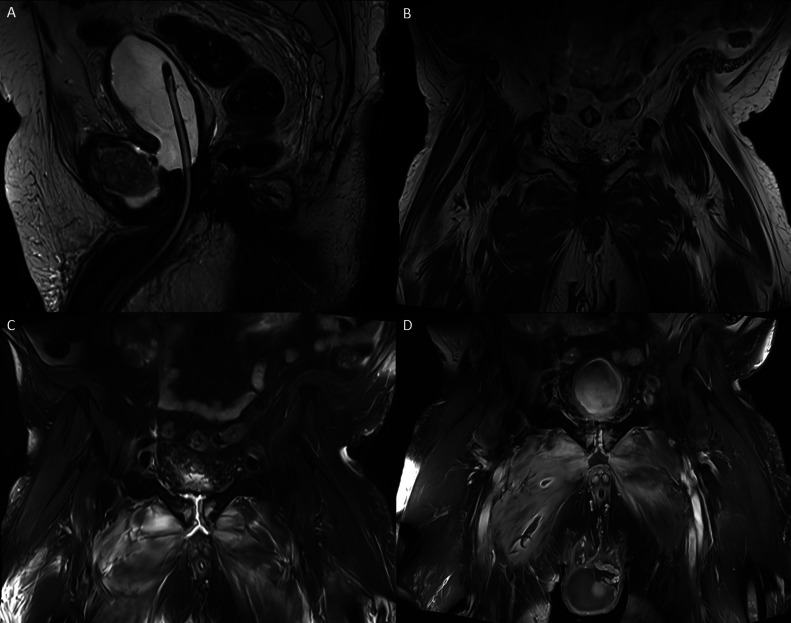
(A) Sagittal T2-weighted turbo spin echo MRI slice demonstrating direct communication between the anterior-inferior bladder and pubic symphysis. (B-D) Coronal MRI slices showing diastasis of the pubic symphysis with low signal intensity on T1 (B), high signal intensity on T2 (C), and enhancement on postcontrast T1 (D) of the medial pubic body margins. These findings are consistent with osteomyelitis. Additional features demonstrate bilateral abductor compartment myositis characterized by T1 isointensity (B) and T2 hyperintensity (C).
